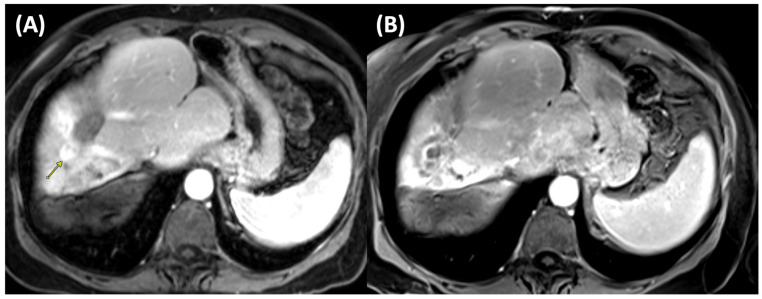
Pre- and post-treatment MRI after radiofrequency ablation (RFA). (A) Pre-treatment MRI shows a 1.4 cm arterially enhancing right hepatic tumor with washout, consistent with hepatocellular carcinoma (arrow). (B) Post-treatment MRI demonstrates no residual arterial enhancement. A thin rim of peripheral T1 hyperintensity surrounds the ablation cavity, representing expected post-procedural change.
